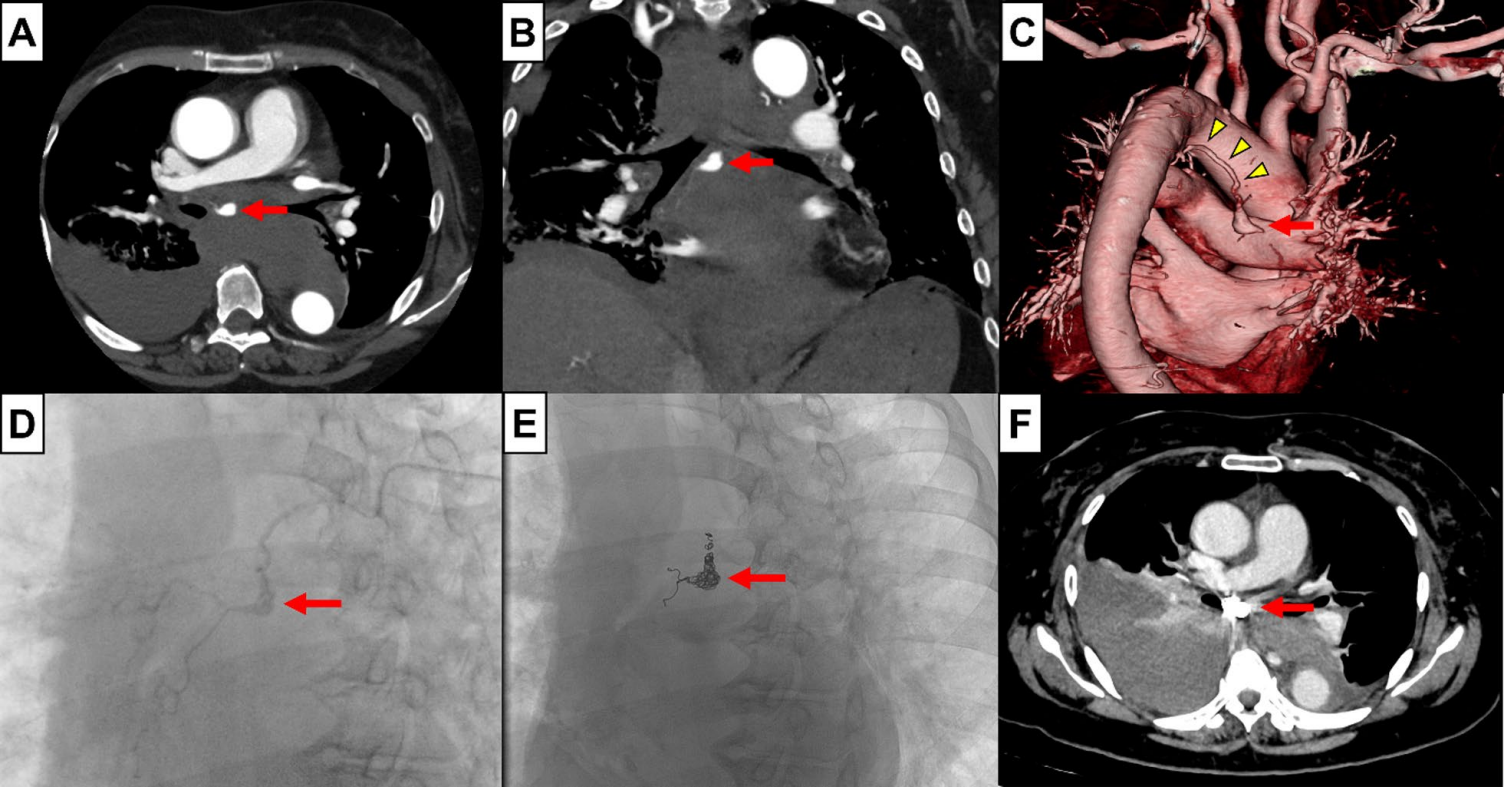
Imaging of the mediastinum. Computed tomography (CT) in the (A) axial and (B) sagittal planes shows a mediastinal BAA (red arrow) with an associated hematoma and right hemothorax. C Three-dimensional CT showing the long neck (yellow arrowheads) of the BAA (red arrow) and its origin from the descending aorta. D Bronchial arteriography showing a BAA (red arrow) in the right bronchial artery. E The BAA (red arrow) is successfully treated with catheter and coil embolization. F Subsequent CT image showing the treated BAA (red arrow) and expanded hemothorax. *BAA* bronchial artery aneurysm, *CT* computed tomography
